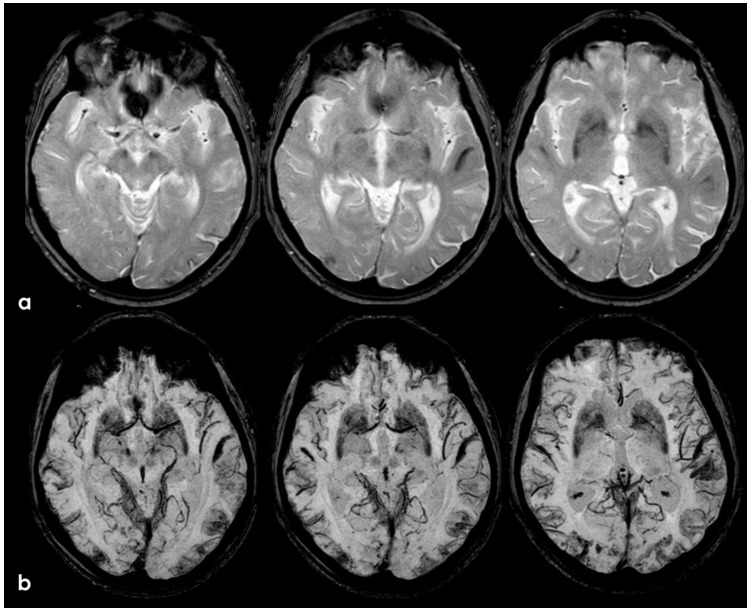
GRE and SWI-MRI (panel (a) and (b), respectively) showing the increased extent of cSS in corresponding axial slices (more evident in SWI than in GRE sequences).
